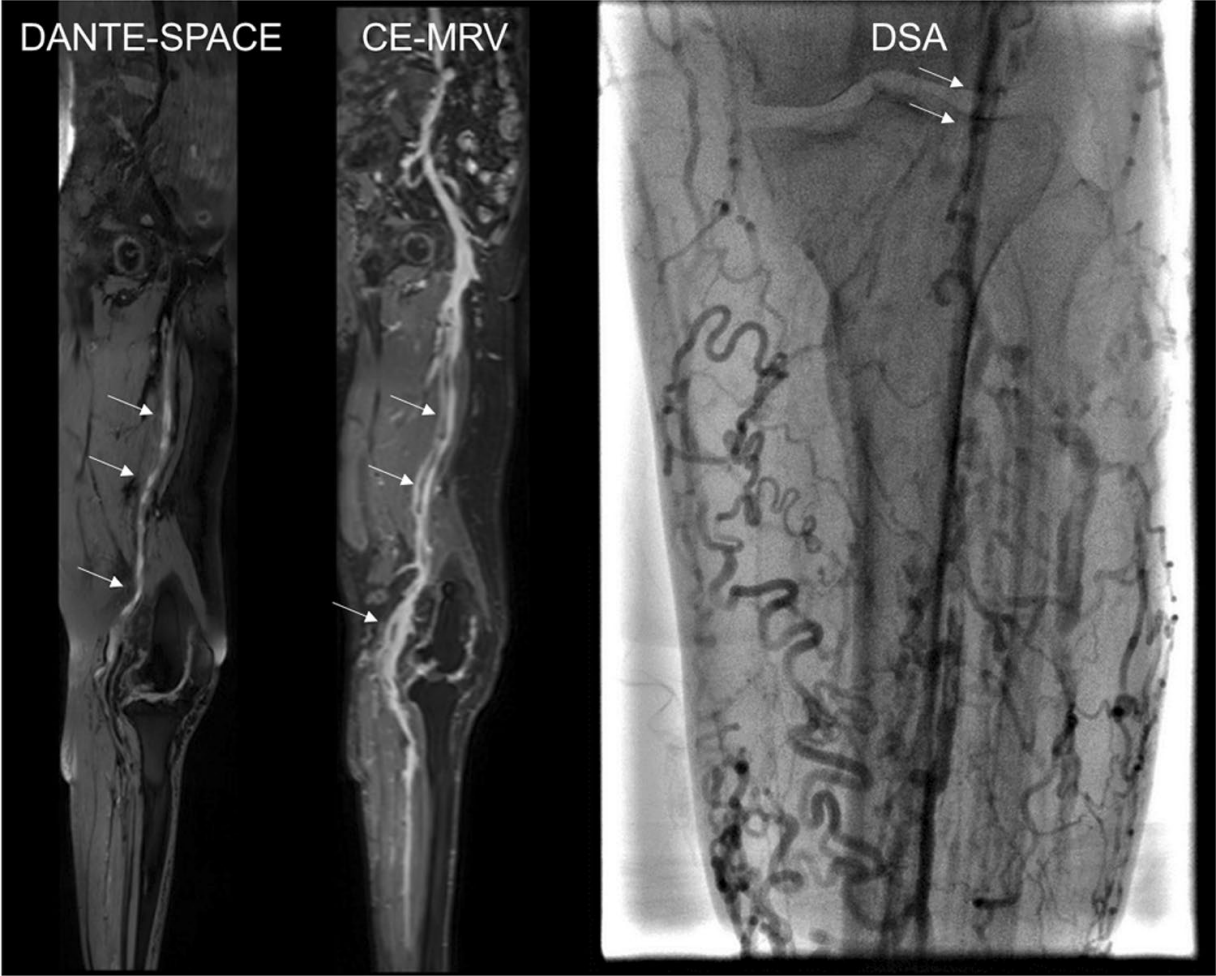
MRI and DSA images of an 85-year-old male with left lower extremity swelling and pain and superficial varicose veins. In the DANTE-SPACE images, the cavity of the common iliac and femoral veins was smooth and showed a low signal. There were multiple thrombi in the middle and lower femoral veins with a high signal at different degrees and rich signal layers, showing signs of a “flow void signal,” and the lesion in the venous vascular wall was rough. In the CE-MRV images, the common iliac and femoral veins were smooth and showed a high signal. In the middle and lower femoral veins, the thrombosis of the femoral vein showed multiple thromboses with a low signal that was characterized by a “filling defect.” The vein wall of the lesion was relatively smooth. In the DSA image, femoral and popliteal occlusion, collateral circulation, and superficial varicose veins were noted
